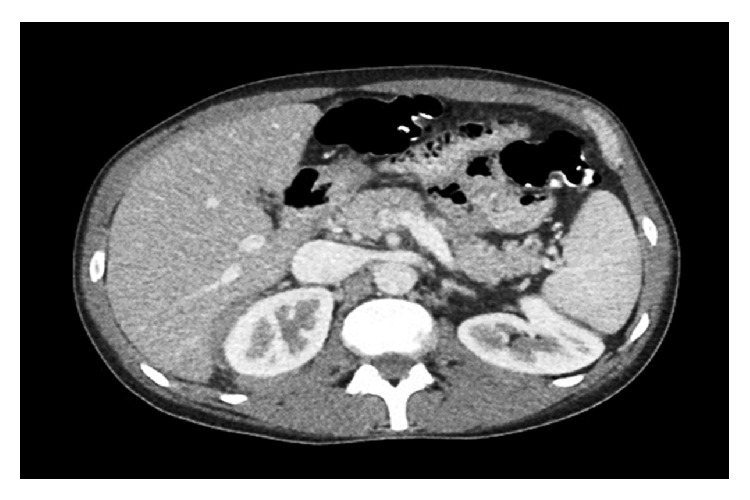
Contrast-enhanced computed tomography at the level of pancreas after 3 months of cyclophosphamide, doxorubicin, vincristine, and prednisone (CHOP) treatment demonstrating near complete resolution of previously seen soft tissue masses.
